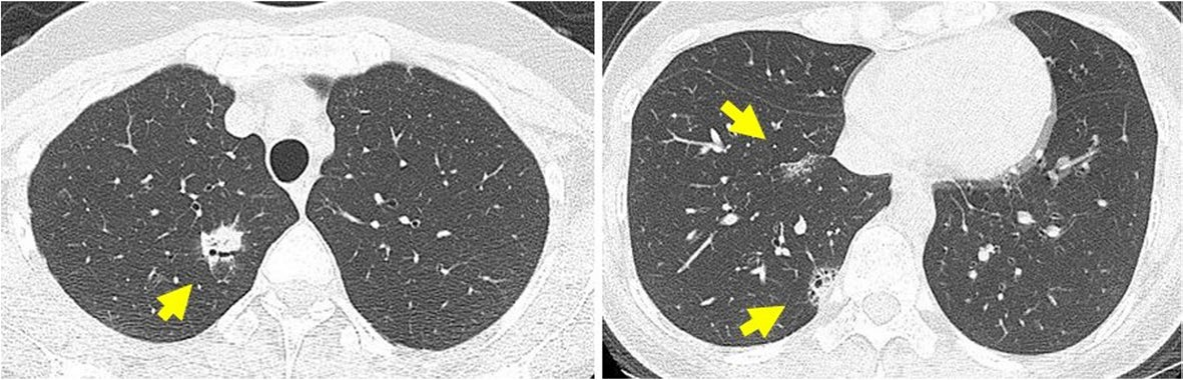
a An infiltrative shadow with an air bronchogram in the right S2 segment. b CT image showing ground-grass opacity and cystic components in the shadows of the right S8 and S10 segment
